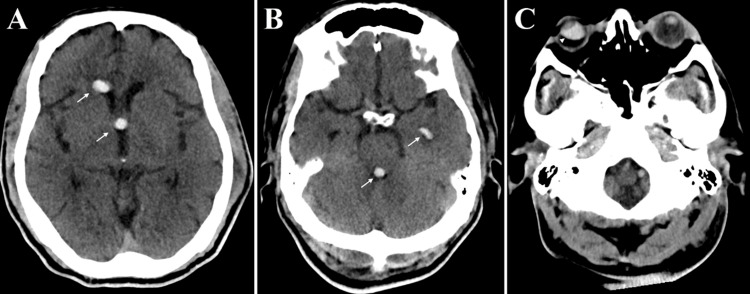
Axial slices of noncontrast head computed tomography demonstrate hyperdense ovoid lesions (white arrows) in the right frontal horn and the left temporal horn of the lateral ventricles, third ventricle, and fourth ventricle (A, B) without evidence of hydrocephalus. Imaging through the orbits shows postoperative changes of a right vitrectomy (white arrowhead, C)
